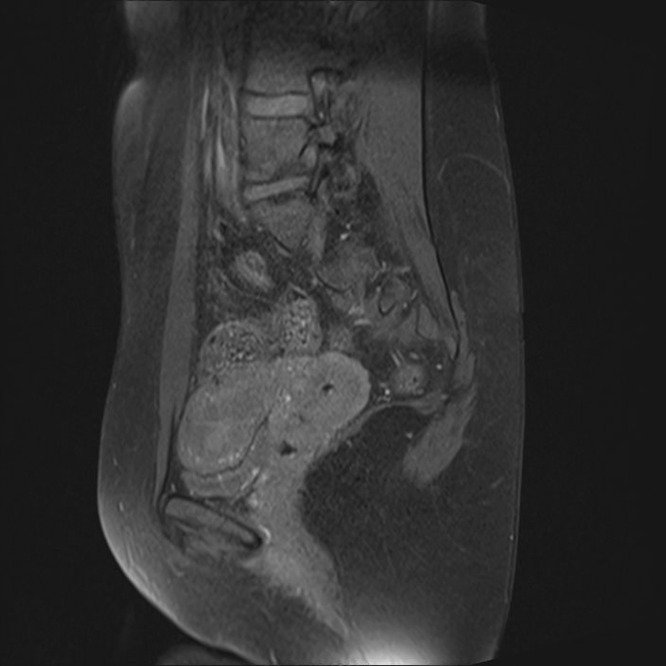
Case 2—Postoperative MRI showing a small linear contrast enhancement at the excision site, unlikely representing residual disease
